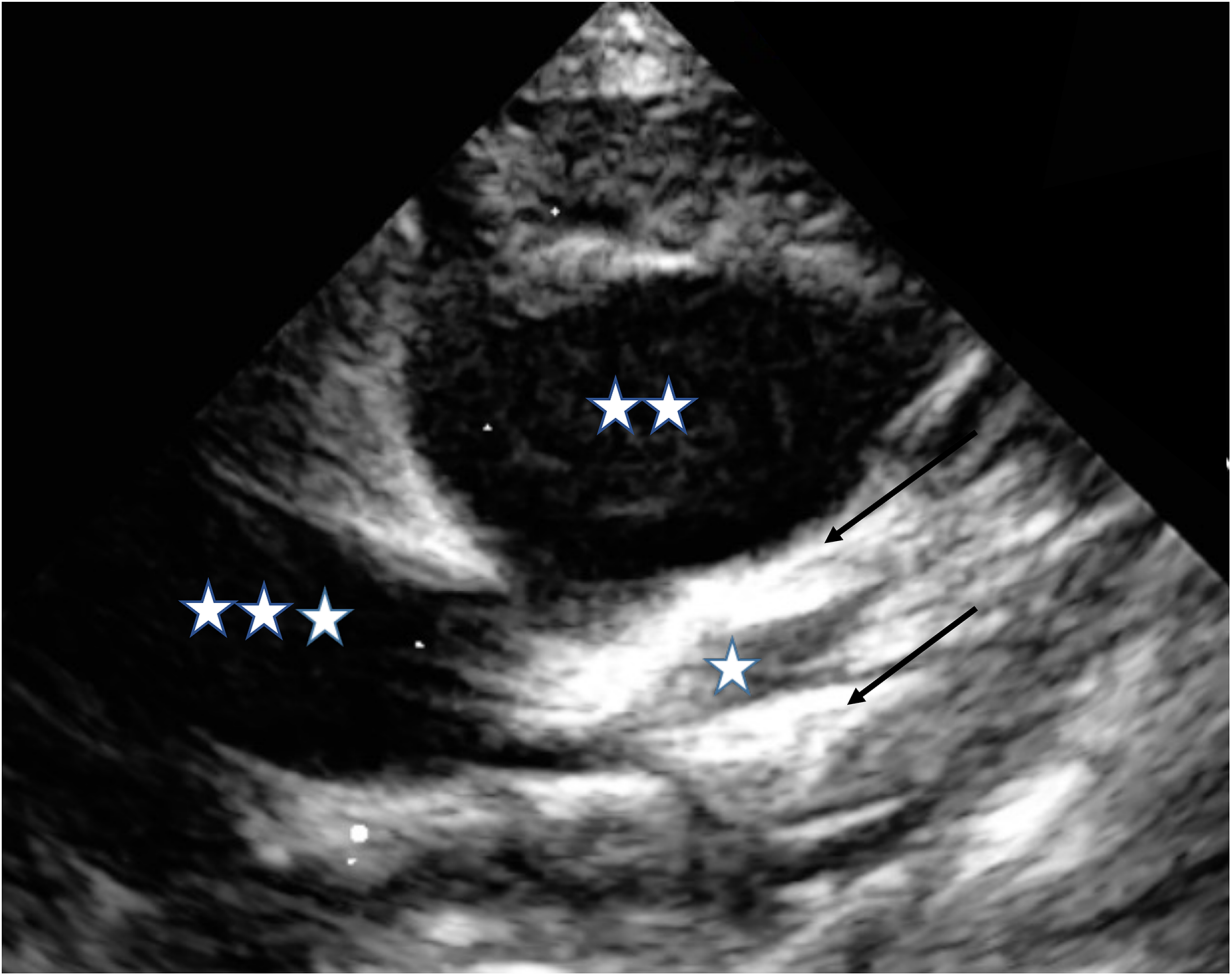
“Bright” appearing perivascular tissue in the echocardiography around CAA. *LAD, left coronary artery; PA, pulmonary artery; *AO, aorta; arrow; “bright” appearing perivascular tissue.
